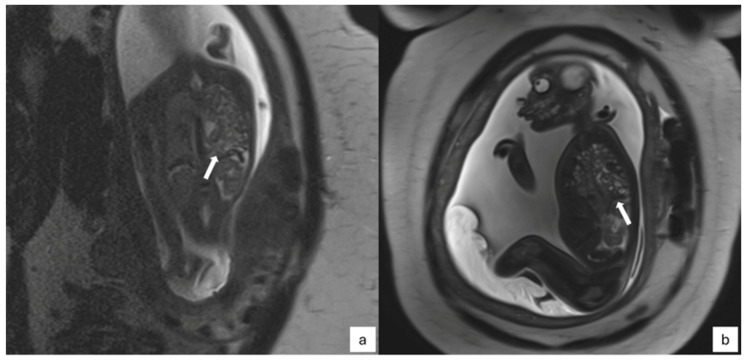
T2-weighted half-Fourier acquisition single-shot turbo-spin echo (HASTE) images of the same fetus with a congenital diaphragmatic hernia (white arrows) in different gestational ages on coronal planes ((a)—23 weeks of gestation) and sagittal planes ((b)—31 weeks of gestation).
